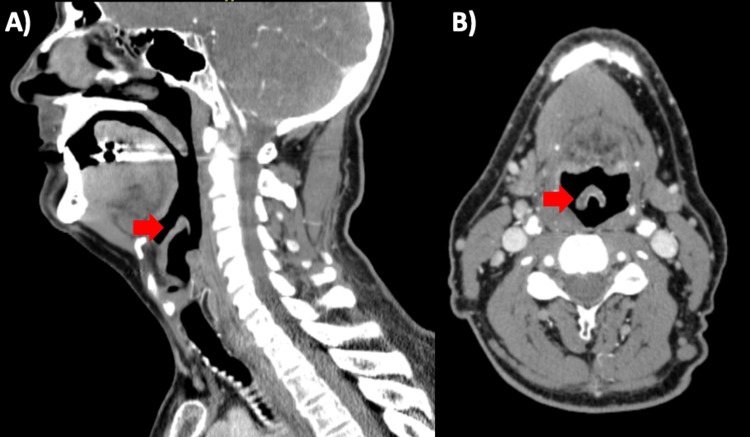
CT imaging of the head and neck depicts asymmetric swelling of the epiglottis (red arrows) in the sagittal (A) and axial (B) planes, most prominent on the right. CT: computed tomography
